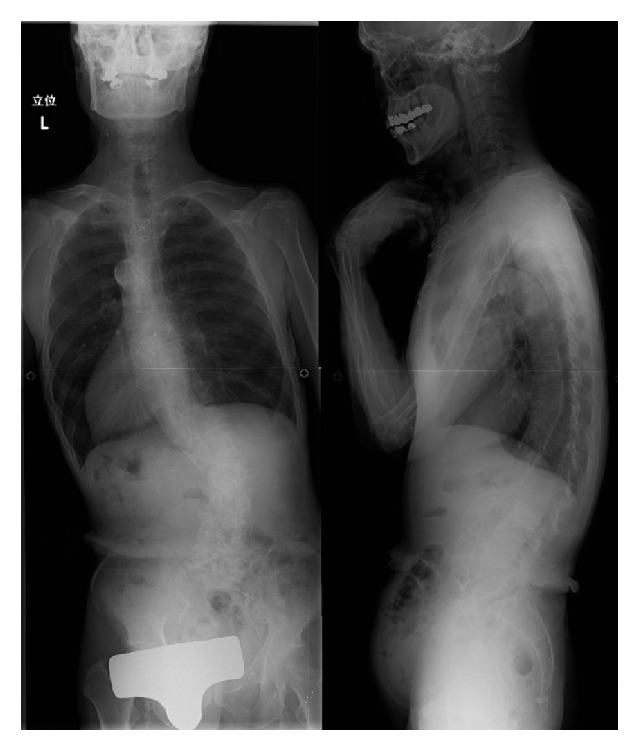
Preoperative radiographs showing right convex lumbar curvature and left-side coronal imbalance.
